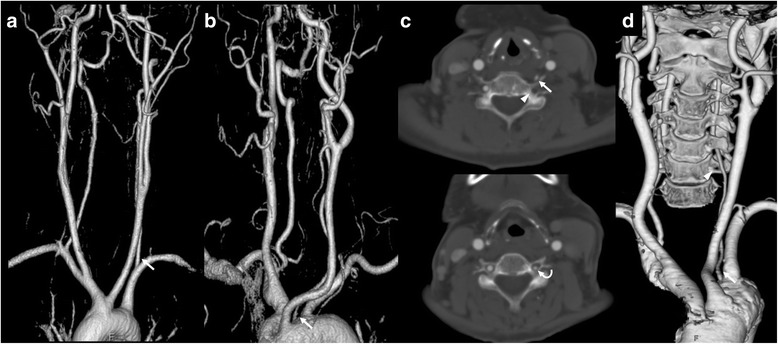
Left VAs (*arrows*) originating from (a) the subclavian artery and (b) the aorta on a three-dimensional MR angiography. Axial source images (window level = 400 Hounsfield Units, width = 1400 Hounsfield Units) of the CT angiography showing that the left VA (c, *arrow*) did not penetrate the C6 transverse foramen (*arrow head*) but that it passed through the C5 transverse foramen (c, *curved arrow*). Three-dimensional reconstructed CT image (d) showing a left VA (*arrow*) with an aortic origin and an empty left C6 transverse foramen (*arrow head*)
